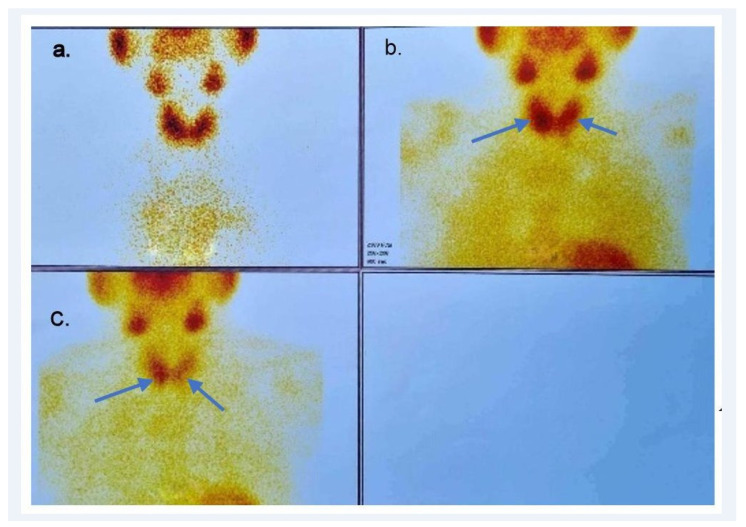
(a) 99Tc; (b) early 99mTc-methoxyisobuthylisonitrile (99mTc-MIBI); (c) delayed 99mTc-MIBI scintigraphy images of the neck: increased radiotracer uptake in the lower third of the right thyroid lobe and posterior to the left thyroid lobe, interpreted as a right inferior and a left inferior parathyroid adenoma, respectively (arrows).
